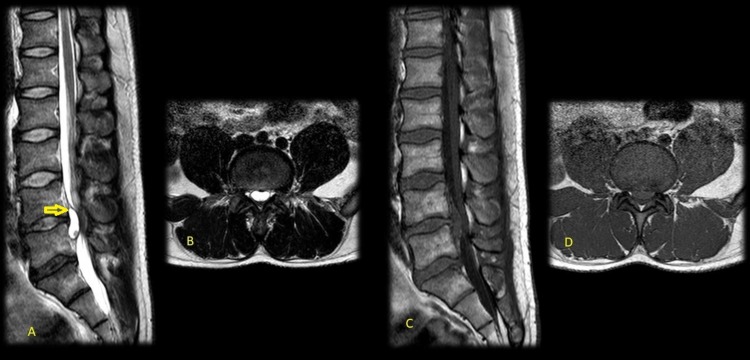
Preoperative Magnetic Resonance Imaging of the Lumbar spine A: Sagittal T2 sequence, B: Axial T2 Sequence, C: Sagittal T1 sequence D: Axial T1 sequence This magnetic resonance imaging sequence shows a lesion (yellow arrow) that is hyperintense on T2 sequences and isointense on T1, with contents that resemble spinal fluid.
